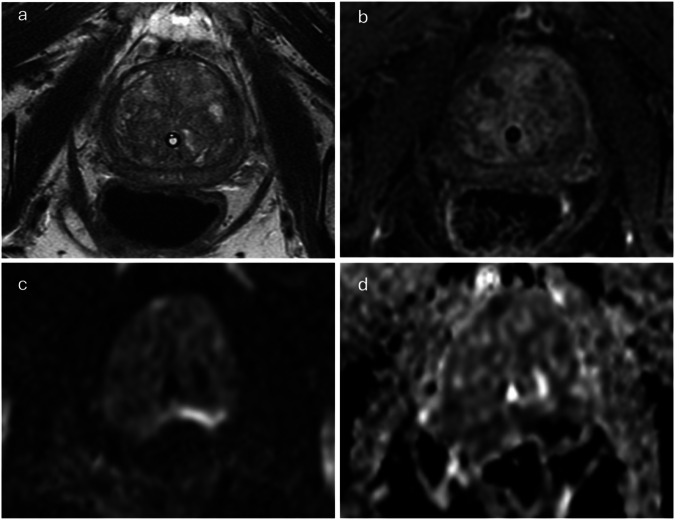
Image quality categorisation varied between versions because of DCE, overall image quality classified as optimal on PI-QUAL v1, inadequate on v2 bpMRI, and acceptable on v2 mpMRI. Image of a 77-year-old patient scanned at 3-T MRI with a presenting PSA of 10.48 ng/mL and a prostate volume of 58.5 cc (PSA density: 0.18 ng/mL/mL). According to PI-QUAL v1, the axial T2WI (a) and DCE (b) sequences exhibit optimal quality, while DWI (c) and ADC (d) were degraded due to susceptibility artefact, particularly at the left posterior aspect of the prostate, resulting in an overall v1 quality score of 4/5, equivalent to optimal image quality (3/3) on a 3-point scale. In contrast, in PI-QUAL v2, T2WI receives full marks (4/4), while DWI loses marks for “artefacts” and “anatomical matching of the ADC map/high *b* values sequences to the axial T2WI”, resulting in a quality score of 2/4 for DWI. Thus, the quality categorisation of bpMRI based on PI-QUAL v2 is deemed inadequate (1/3). However, because DCE receives full marks (2/2) according to PI-QUAL v2, the overall quality of mpMRI is upgraded to an acceptable level of image quality (2/3)
